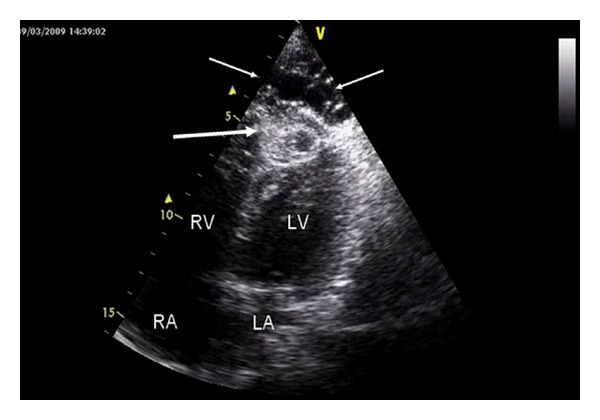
Apical four chamber view, revealing multiple hydatid cysts. Multiple small cysts surrounding and obliterating the apical region (small arrows). Relatively bigger cyst invasing the apicoseptal region (big arrow).
